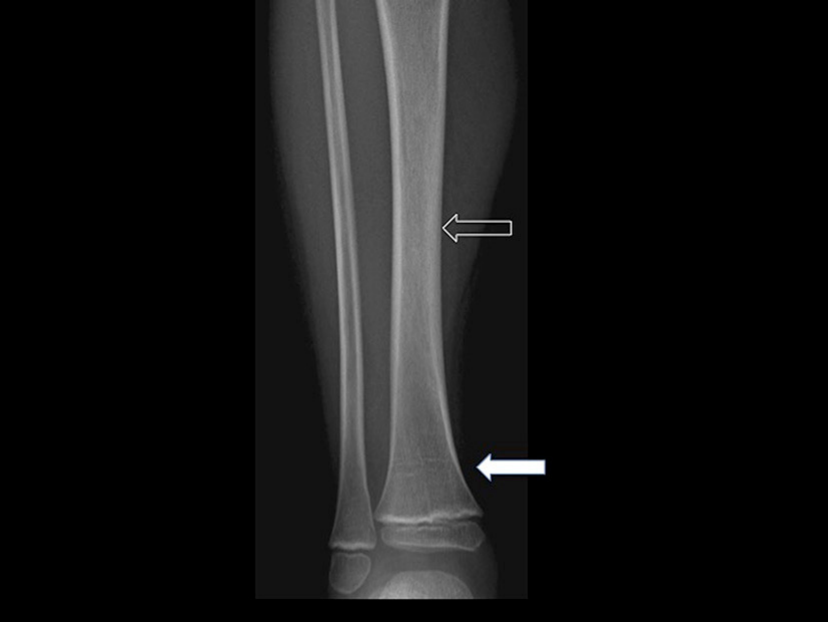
Frontal radiograph of right leg demonstrates multiple transverse growth recovery lines (solid arrow) and inhomogeneous density of tibial diaphysis (open arrow). These findings were not initially appreciated.
